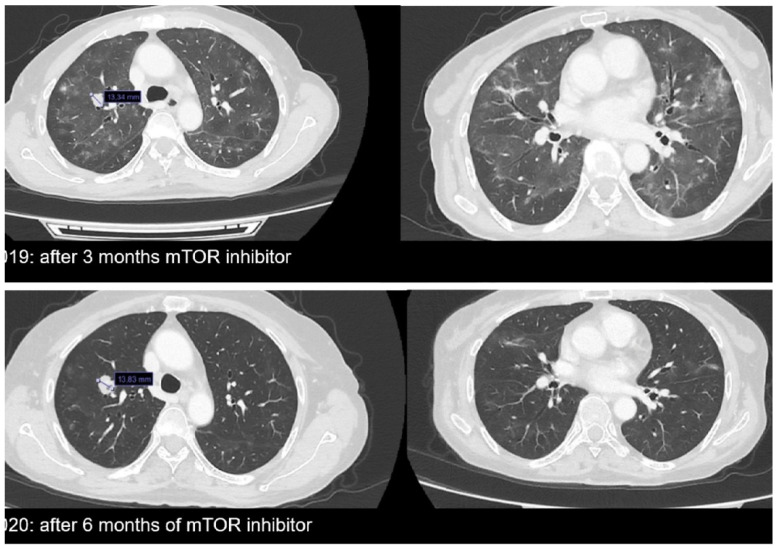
Computed tomography imaging in October 2019 and January 2020 during mTOR-inhibitor treatment.
